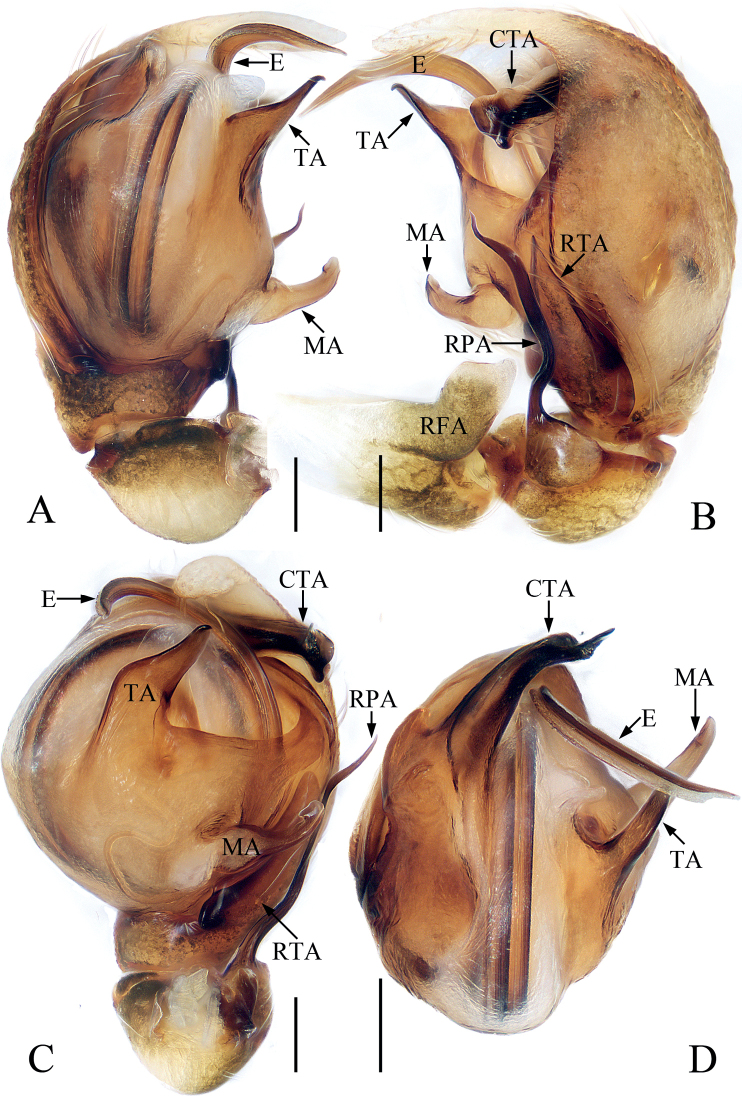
Male palp of *Eupoamaidinhyeni* sp. nov., holotype and paratype A holotype, prolateral B ditto, retrolateral C ditto, ventral D paratype bulb, apical. Scale bars: 0.1 mm. Abbreviations: E – embolus; CTA – compound terminal apophysis; MA – median apophysis; RFA – retrolateral femoral apophysis; RPA – retrolateral patellar apophysis; RTA – retrolateral tibial apophysis; TA – terminal apophysis.
